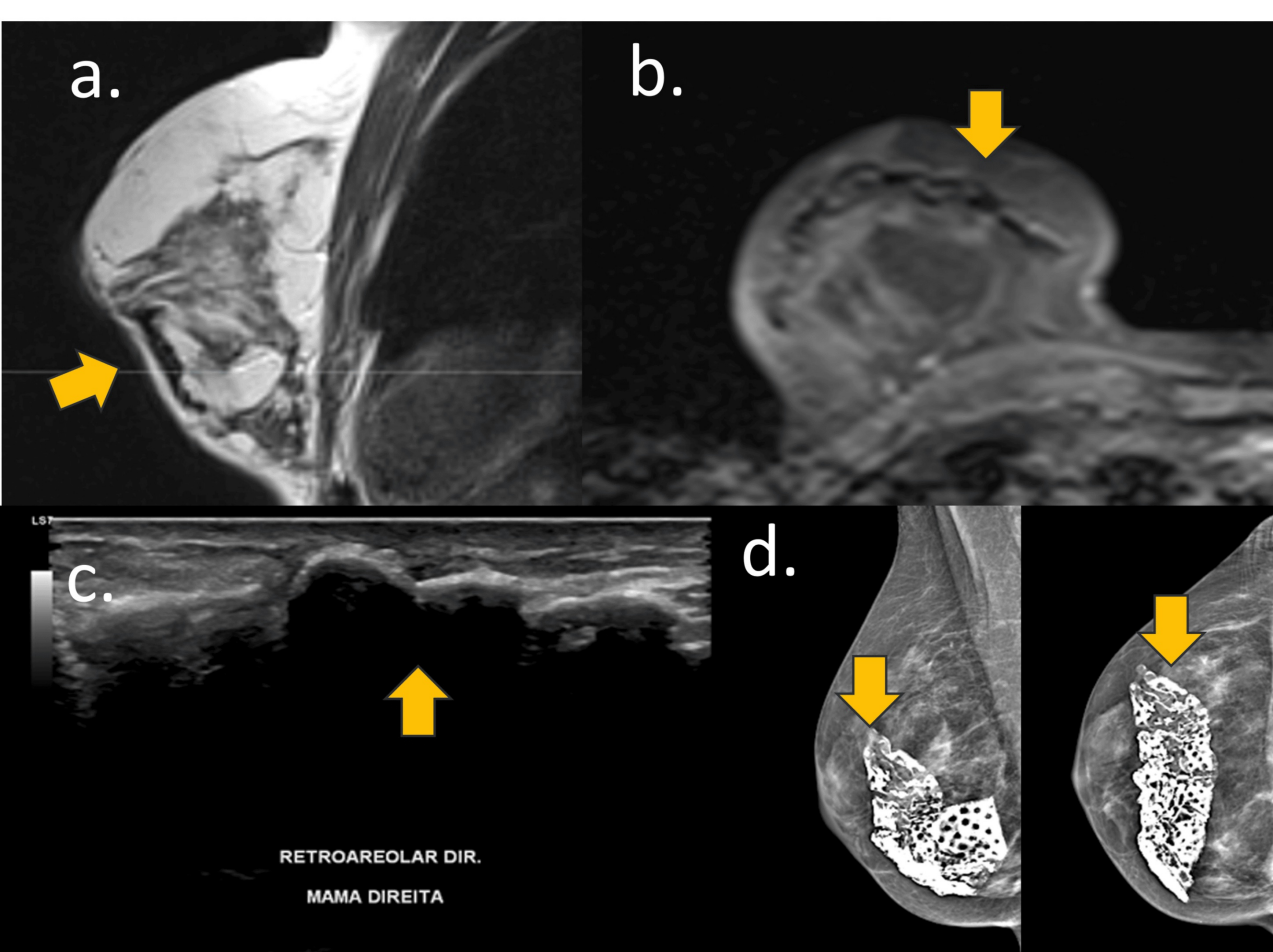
Marlex mesh A 67-year-old woman with a retropectoral implant and Marlex mesh for 30 years. (a) Sagittal DP-weighted sequence, (b) axial T1-weighted sequence, (c) breast USG, and (d) mammography An example of Marlex mesh during mastopexy 30 years ago. (a and b) Marlex mesh appears as a hypointensity signal on MRI, (c) hyperechoic mass with acoustic shadow on USG, and (d) calcified foreign body on mammography.
